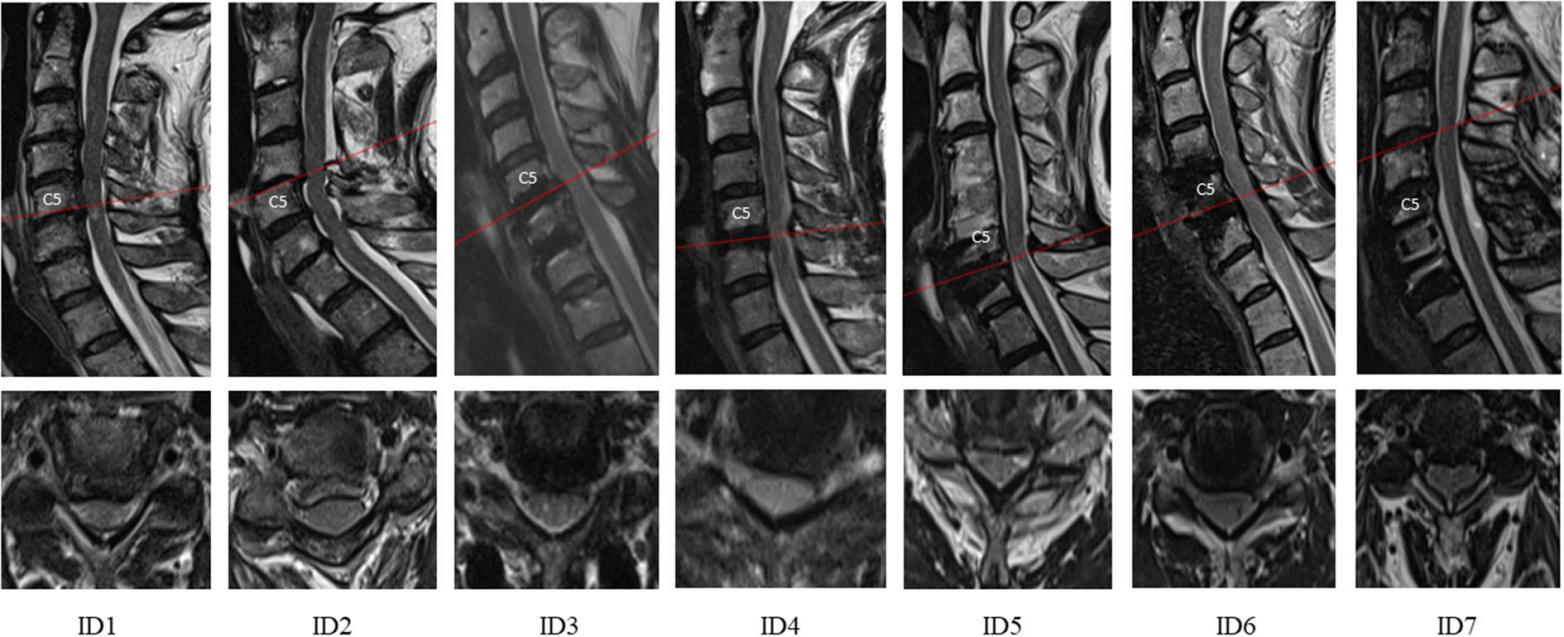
Sagittal and axial cervical MRI from the time of consultation shown for all subjects (ID1-7). The red line indicates the level of maximum stenosis. Spine imaging shows that all patients had spinal canal stenosis; T2-hyperintensity was present in 5 out of 7. Structural changes from previous spine surgery can be seen (ID3-7, e.g., anterior decompression with fusion in ID7).
